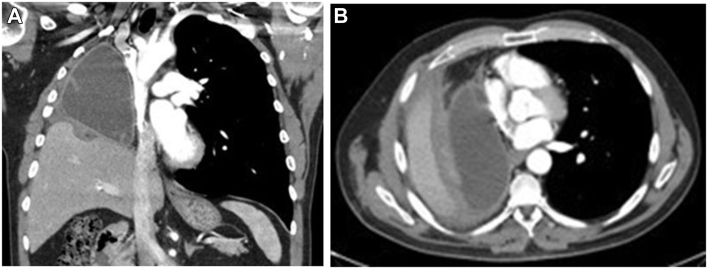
Postoperative chest computed tomographic scans. (A) Coronal image shows the advantageous position of the tissue expander with reduction of the diaphragm. (B) Axial image shows the tissue expander and improved cardiac silhouette, without compression.
